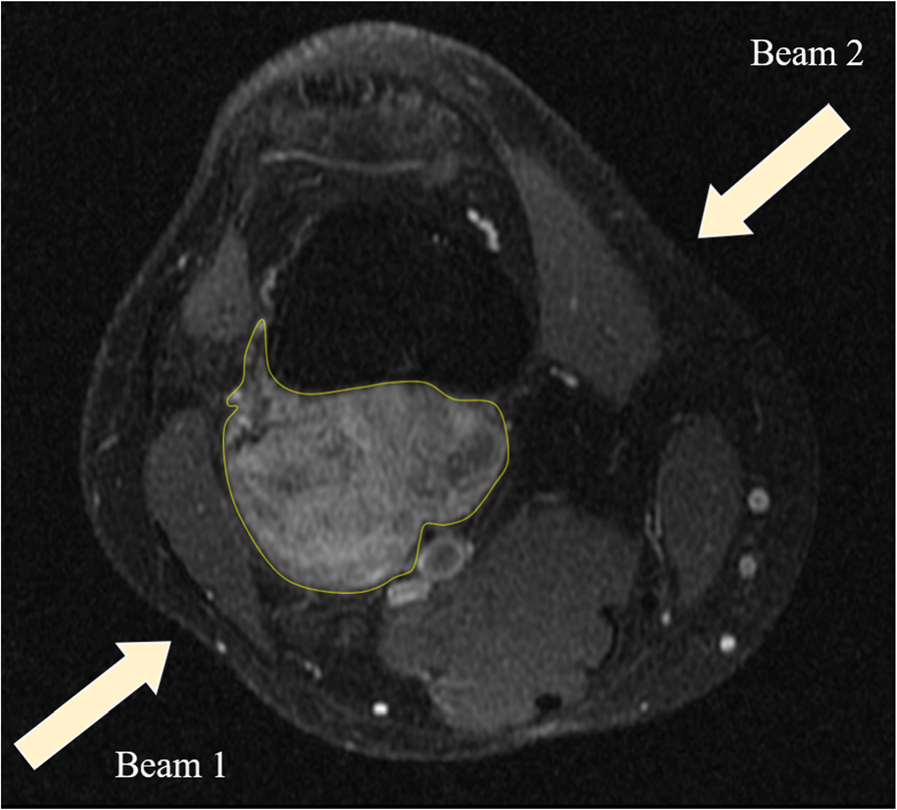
MRI image of the osteosarcoma tested with the selected beam configuration
